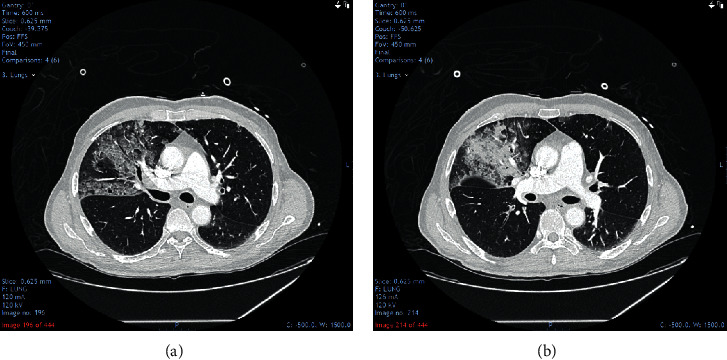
CT pulmonary angiogram report: there are no pulmonary emboli and pleural or pericardial effusions. There is consolidation within the right upper lobe with subtle peripheral ground glass opacification noted in the left upper lobe and in both lower lobes. Appearances are indeterminate but could represent early COVID-19 disease. The central and peripheral airways are patent with no lower cervical, axillary, and mediastinal lymphadenopathy and lung mass. Other viscera are unremarkable.
